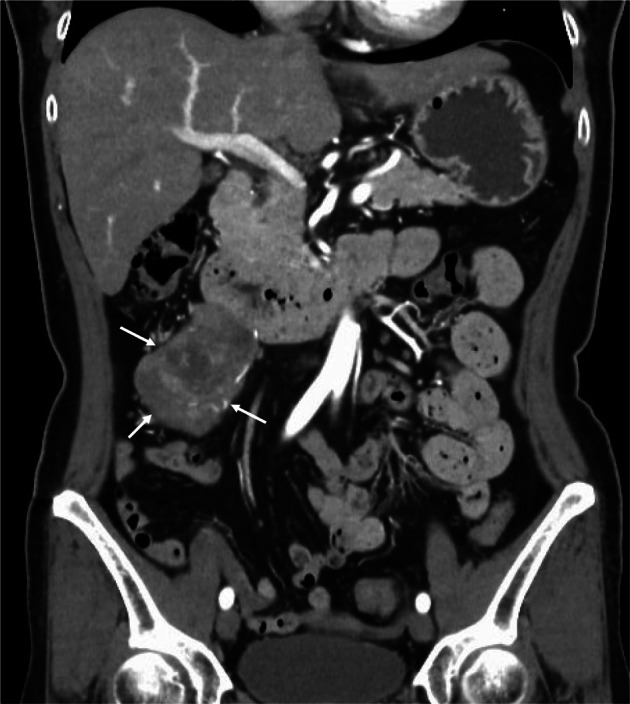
CT findings. CT showed a polygonal tumor with heterogeneous enhancement (arrows) adjacent to the duodenum.
